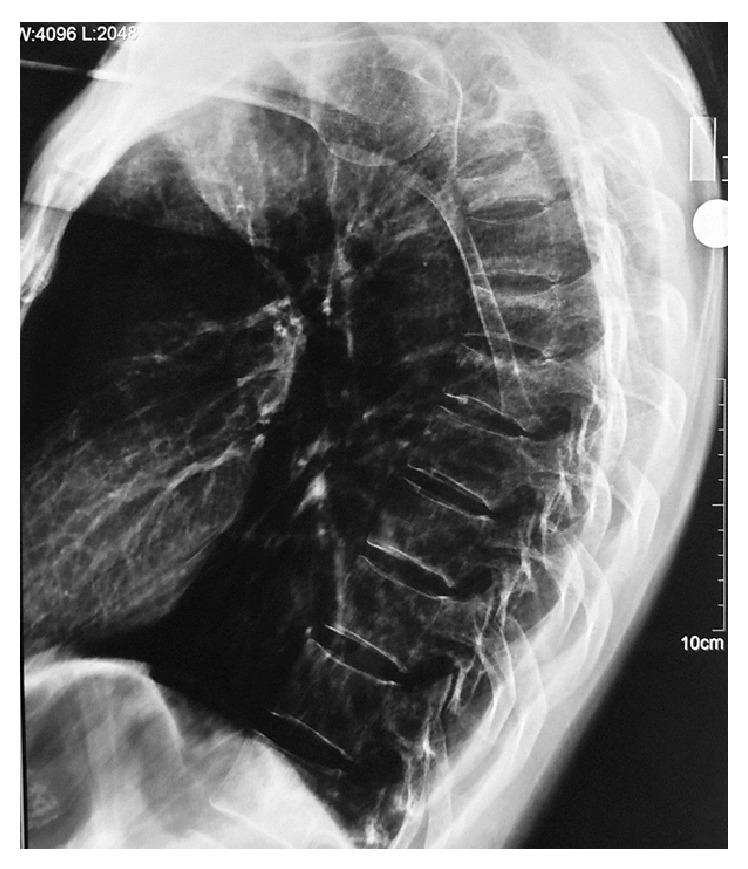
Lateral radiograph of thoracic vertebrae 4 months after the nocturnal convulsion attack.
